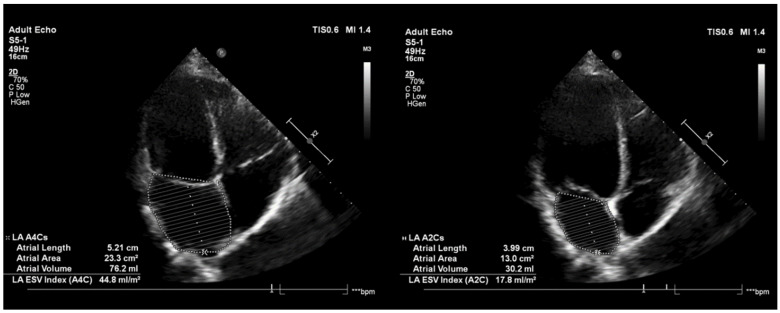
The evaluation of post-procedural LA function by echocardiography.
